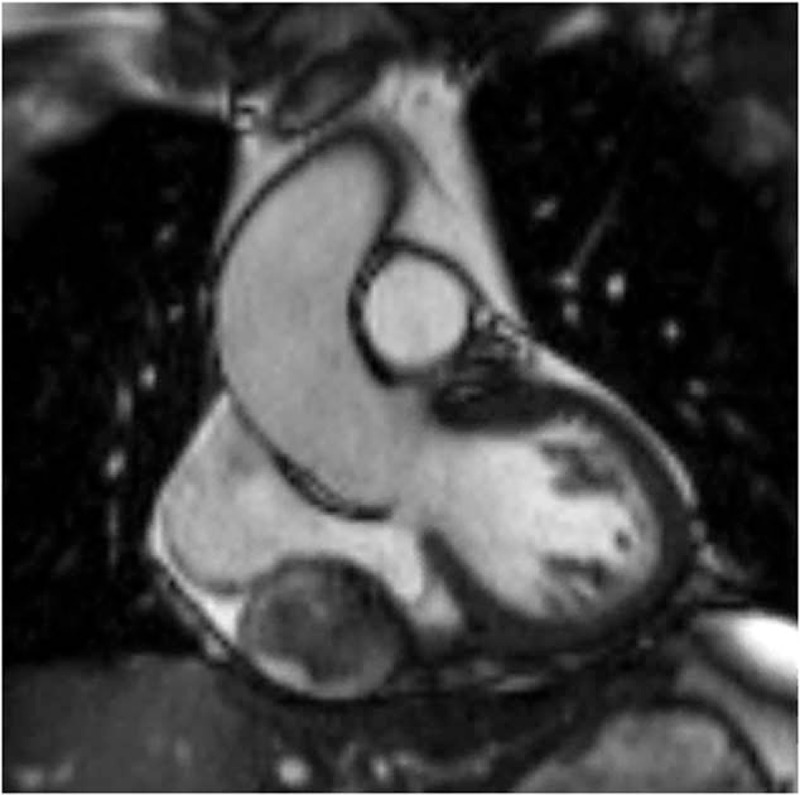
Magnetic resonance imaging depicted a tubular shadow, which compressed the right atrium, under the pericardium adjacent to the right atrioventricular groove, with an internal heterogeneous acoustic signal and smooth edges. Parts of the shadow and the wall showed a delayed enhancement.
